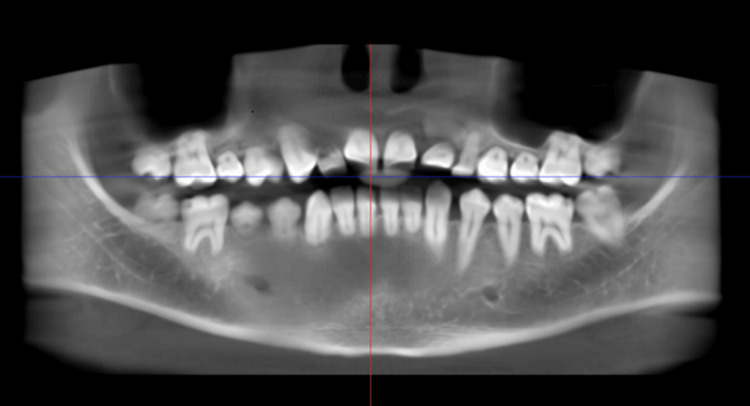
CBCT of the patient showing generalized shortening of roots with normal crowns, which is consistent with dentin dysplasia Image credit: Dr. Gauri Sharma CBCT: Cone-beam computed tomography
